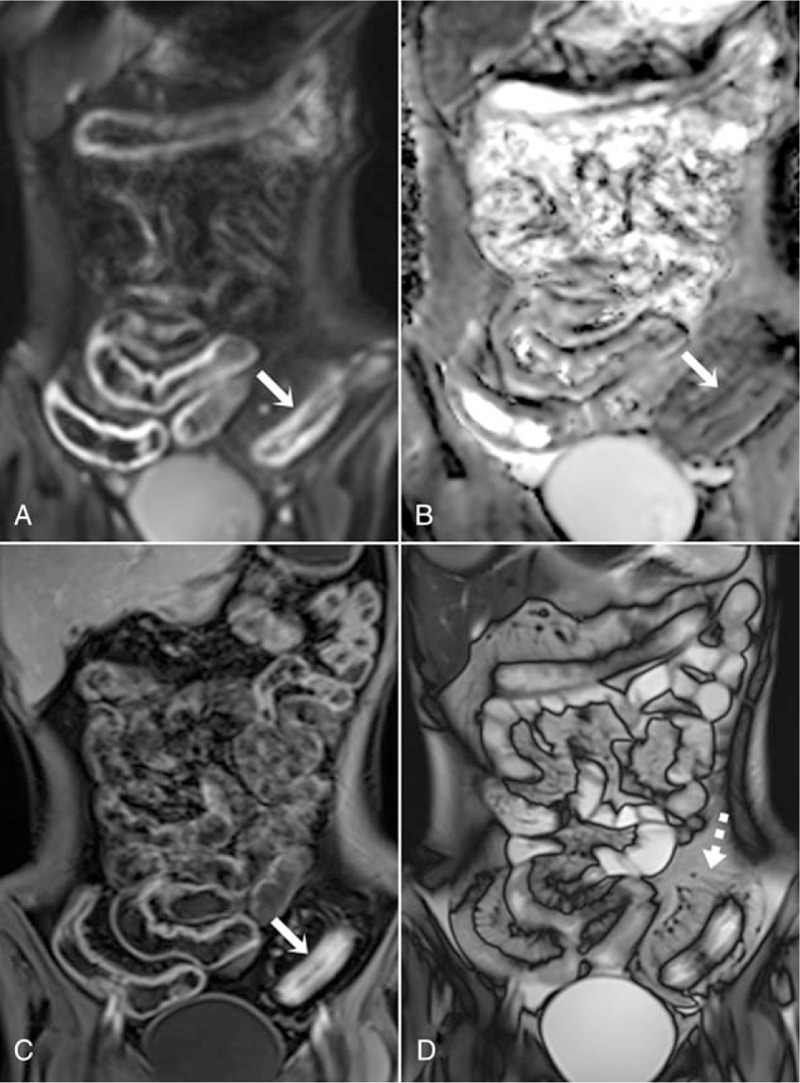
MR enterography images of an 18-yr-old female patient with severe active Crohn disease. Diffusion restriction is observed in the sigmoid colon in the coronal DWI (A) and ADC map (B) (arrows). Because the DWI signal increase is higher than in the lymph nodes, it scored 3 points on the MRAI. On the contrast-enhanced coronal T1-weighted image (C) of the same patient, mural thickening of the sigmoid colon and hyperenhancement that is more apparent in mucosa are seen (arrow). Because contrast enhancement is similar to that of the nearby vascular structures, it was scored as 3 points. Moreover, comb sign is observed on the coronal TRUFI sequence (D) (dashed arrow). ADC = apparent diffusion coefficient, DWI = diffusion weighted imaging, MR = magnetic resonance.
